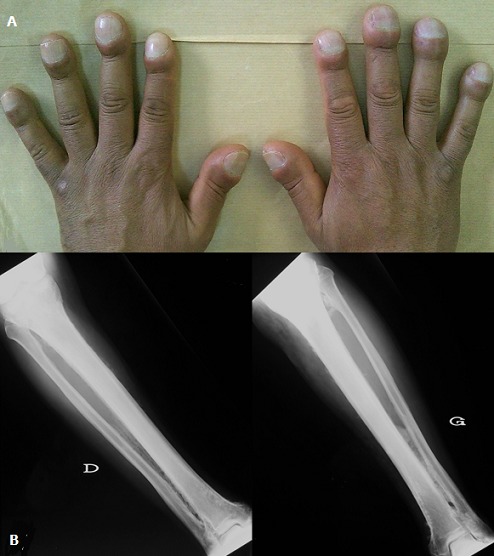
A) digital clubbing (hippocratic fingers); B) radiographs of the tibias and fibulas show bilateral extended and multilayered periosteal thickening
